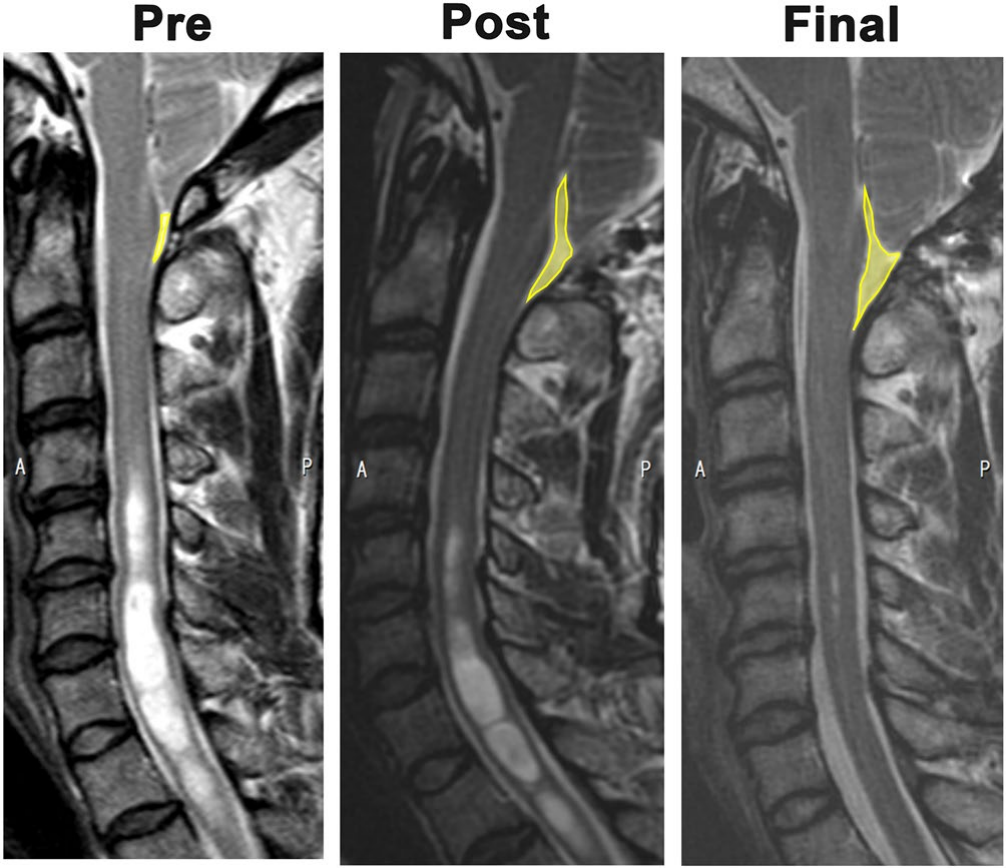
pSAS was defined as the area between the dura mater and spinal cord from the foramen magnum to C2 on a midline sagittal image on T2-weighted imaging. The yellow line represents the outline of the pSAS. Representative MRI image with syrinx improvement. Right, preoperative images; Middle, postoperative images; Left, images at final follow-up.
